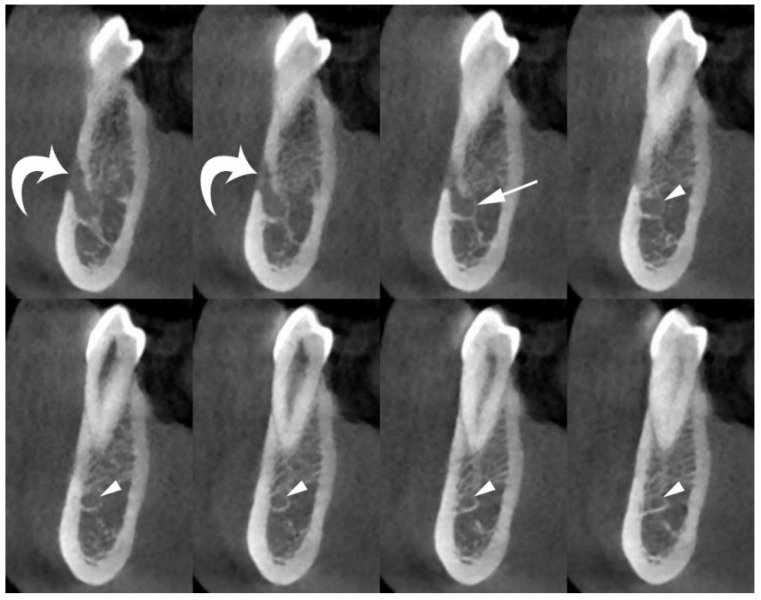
Cross-sectional CBCT images show the origin and position of the MIC (arrowheads) within the spongiosa of the mandible, and its relationships with adjacent anatomical landmarks (cortical plates and first premolar apex). The mental foramen (curved arrows), and anterior loop of mandibular canal (arrow) are also shown.
